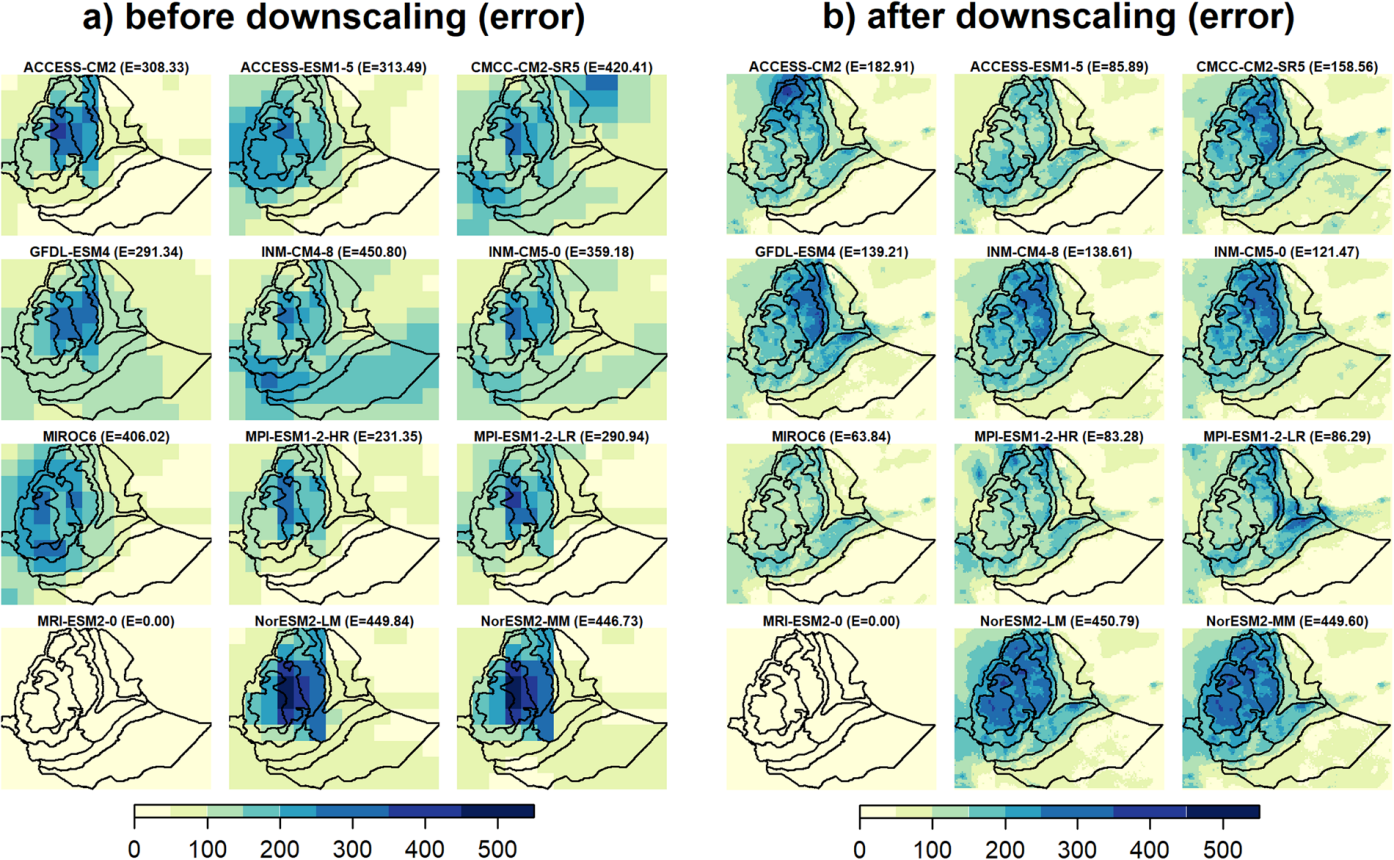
Evaluation (based on RMSE) of GCM outputs using the PS framework (MRI-ESM2-0 selected as a perfect sibling) for JJAS total precipitation (mm) for the 2050s under the SSP3-7.0 scenario, (a) before downscaling and (b) after downscaling. The mean RMSE errors for the whole region are shown in brackets (E) on top of each plot.
